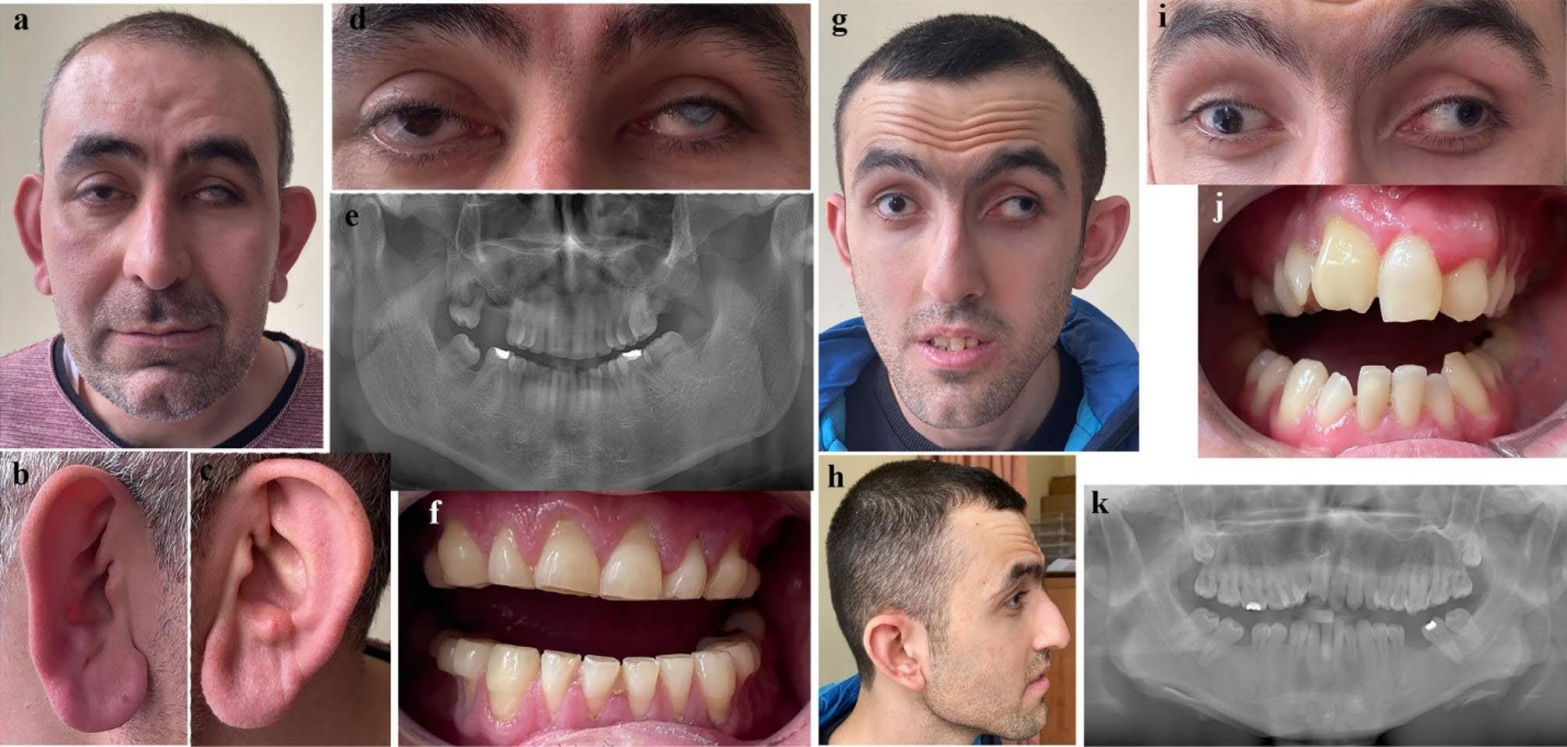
Clinical and radiographic images of affected proband (a-f) and his affected maternal half-brother (g-k) from Family 3. (a-c) Facial and ear close up views showing mild dysmorphic features, including prominent nasal bridge, overfolded and underfolded helix, and prominent and large lobulated ears. (d) Eye close up view demonstrating left corneal opacity and exotropia. (e, f) Intraoral and radiographic images demonstrating severe attrition of all teeth and rounded-shaped molars. (g, h) Frontal and lateral views showing mild dysmorphic features, including a triangular-shaped face with mid-face hypoplasia, arched eyebrows, low-hanging columella, low-set posteriorly rotated right ear, and a prominent left one. (i) Note the small irises and left exotropia (j, k) Intraoral and radiographic images demonstrating screwdriver-shaped incisors, rounded-shaped molars, and supernumerary molars
